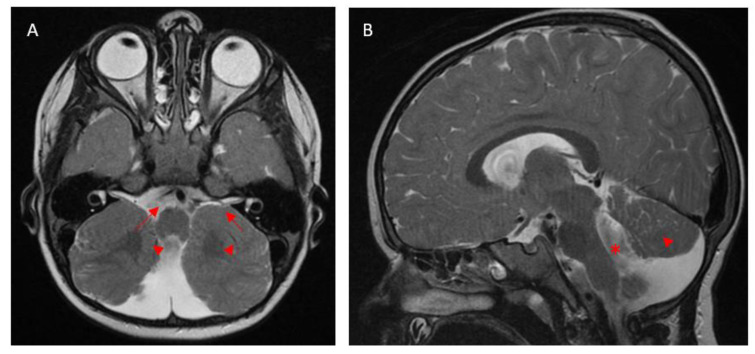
(A) Axial and (B) sagittal views of a T2-weighted MRI brain scan with gadolinium contrast enhancement. The axial view (A) shows hindbrain malformation with severe cerebellar dysgenesis affecting both cerebellar hemispheres. The superior cerebellar peduncles (marked with arrows) are thin and bowed out laterally, and the cerebellar vermis is malformed and hypoplastic (marked with arrowheads). The sagittal view (B) shows vermis hypoplasia (marked with arrowhead) with a widened dysmorphic fourth ventricle (marked with *).
